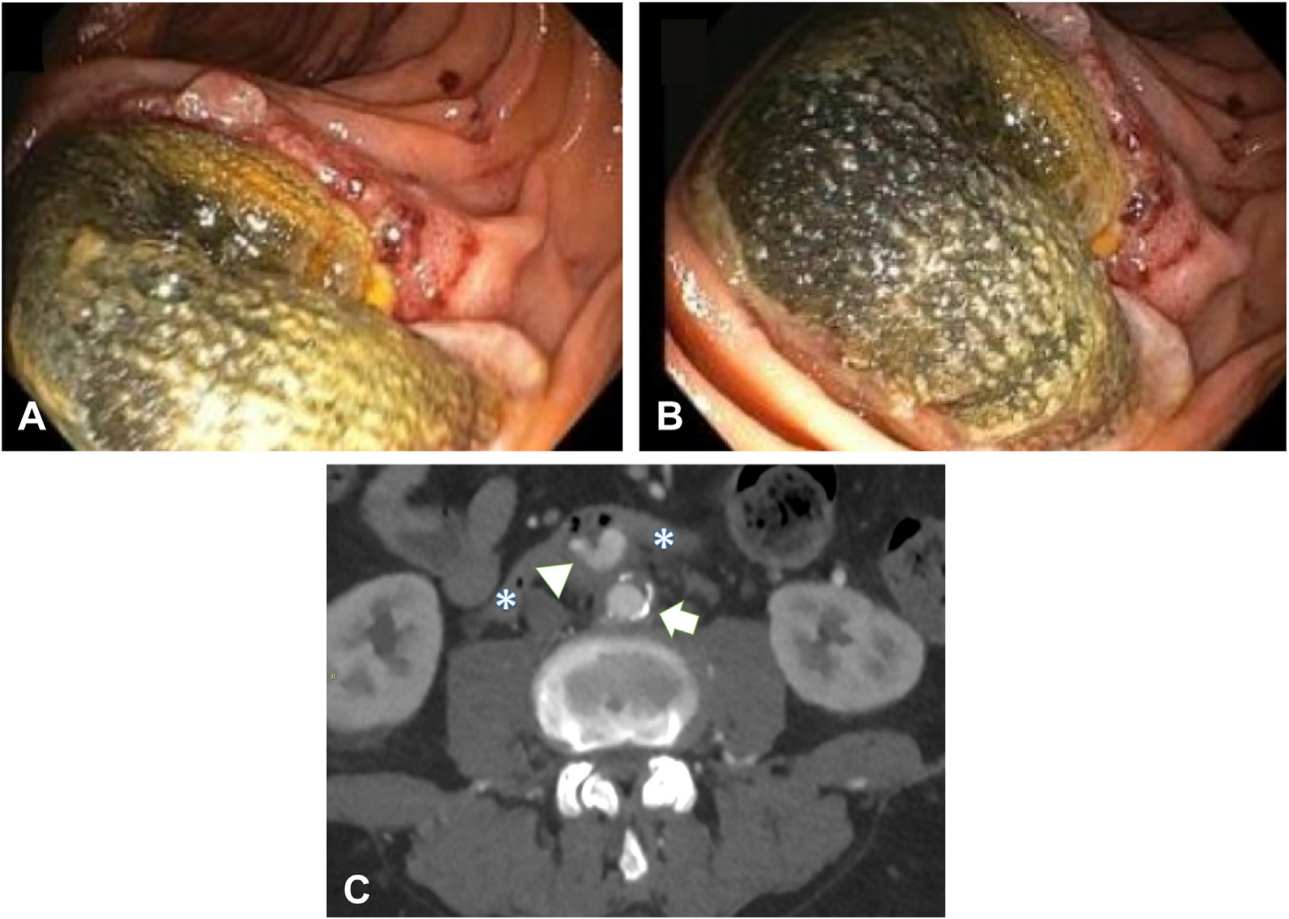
Motorized spiral Endoscopy image of the protruded stent graft. A and B, Motorized spiral enteroscopy showing a graft stent extruding from the duodenum bowel wall. C, CT angiography after injection of contrast material. The graft (*arrowhead*) is seen protruding into the duodenal bowel wall (*asterisks*). Notice the calcified aorta (*arrow*).
